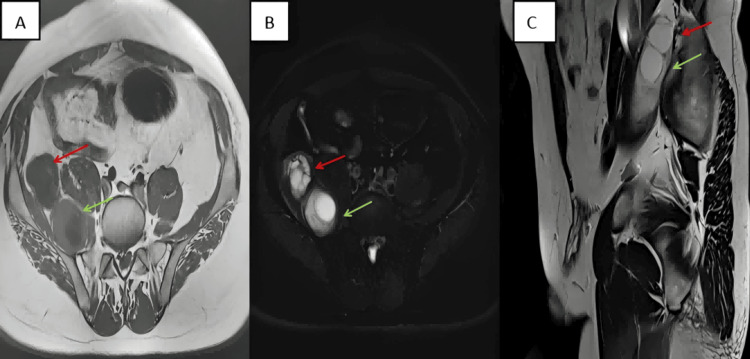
MRI image (A) Axial T1W1 pelvis: superior lesion demonstrates heterogeneous hypointense signal with characteristic cystic signal distribution along the margins(indicated by the red arrow); the inferior lesion shows similar imaging characteristics to the superior lesion(indicated by green arrow). (B) Axial T2W1 pelvis: superior lesion exhibits heterogeneous hyperintense signal with daughter cysts arranged peripherally along the mother cyst wall (red arrow); inferior lesion presents with comparable heterogeneous hyperintense signal (green arrow). (C) Sagittal T2W1 pelvis: superior lesion (red arrow), Inferior lesion (green arrow).
